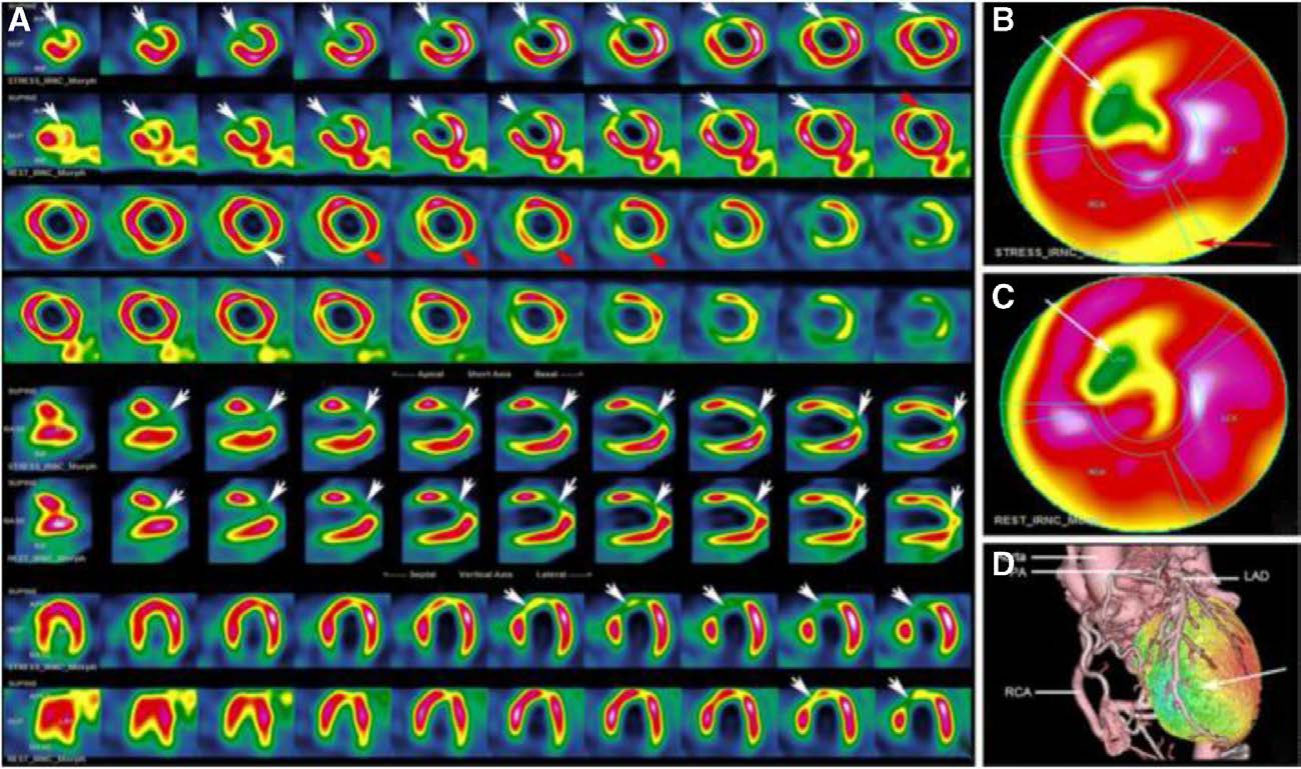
SPECT MPI and fusion images (A) Stress and rest serial images, stress images showed in odd rows and rest images showed in even rows; (B) stress polar map of MPI; (C) rest polar map of MPI; (D) CCTA and surface 3 dimension of stress MPI fused image. SPECT MPI showed fixed perfusion defects in the part of apical segment, apical and middle segments of the anterior wall, and the apical segment of anteroseptal wall (A, B, C and D, as indicated by the white arrows), with a small area of reversible defect in the inferior wall (A and B, as indicated by the red arrows).^[11]^
